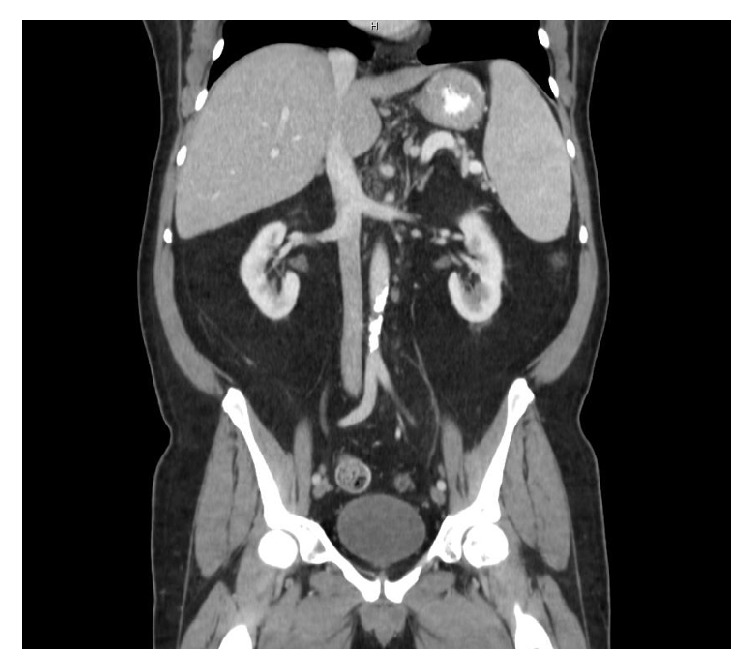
Posttreatment CT with contrast of the abdomen showing resolution of the enlarged intra-abdominal lymph nodes and reduction in splenic size.
